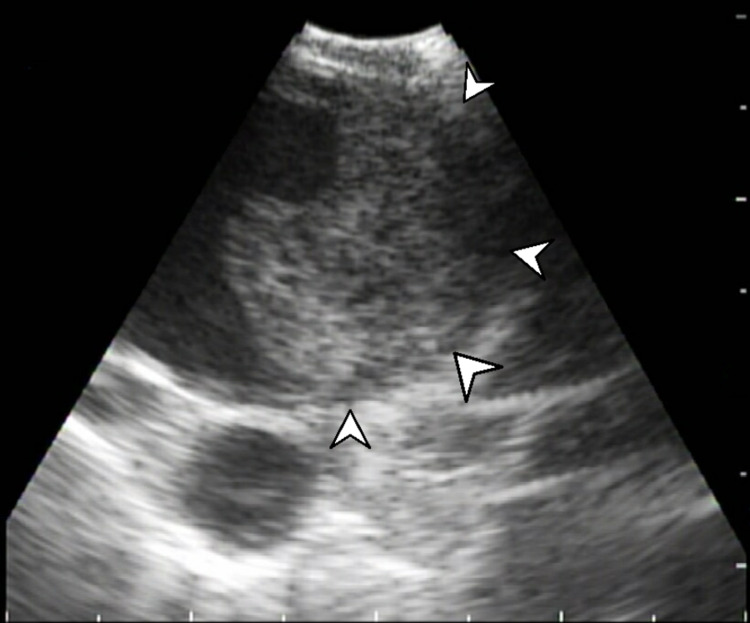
EBUS imaging of the 11R station, demonstrating a newly discovered pulmonary embolism (white arrowheads) EBUS: endobronchial ultrasound
